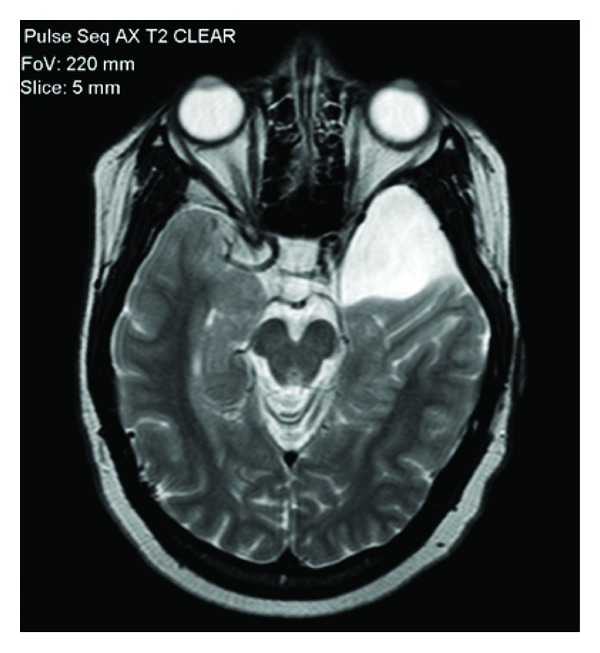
Cranial MRI without contrast in the horizontal plane, demonstrating the mass effect on the left temporal lobe by the arachnoid cyst.
